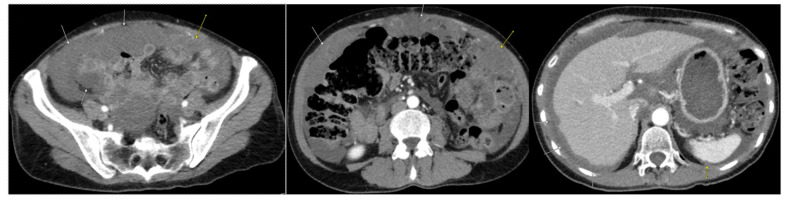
Initial CT scan of abdomen/pelvis completed at time of diagnosis. Arrows indicate diffuse mucinous peritoneal metastases throughout the abdomen and pelvis.
